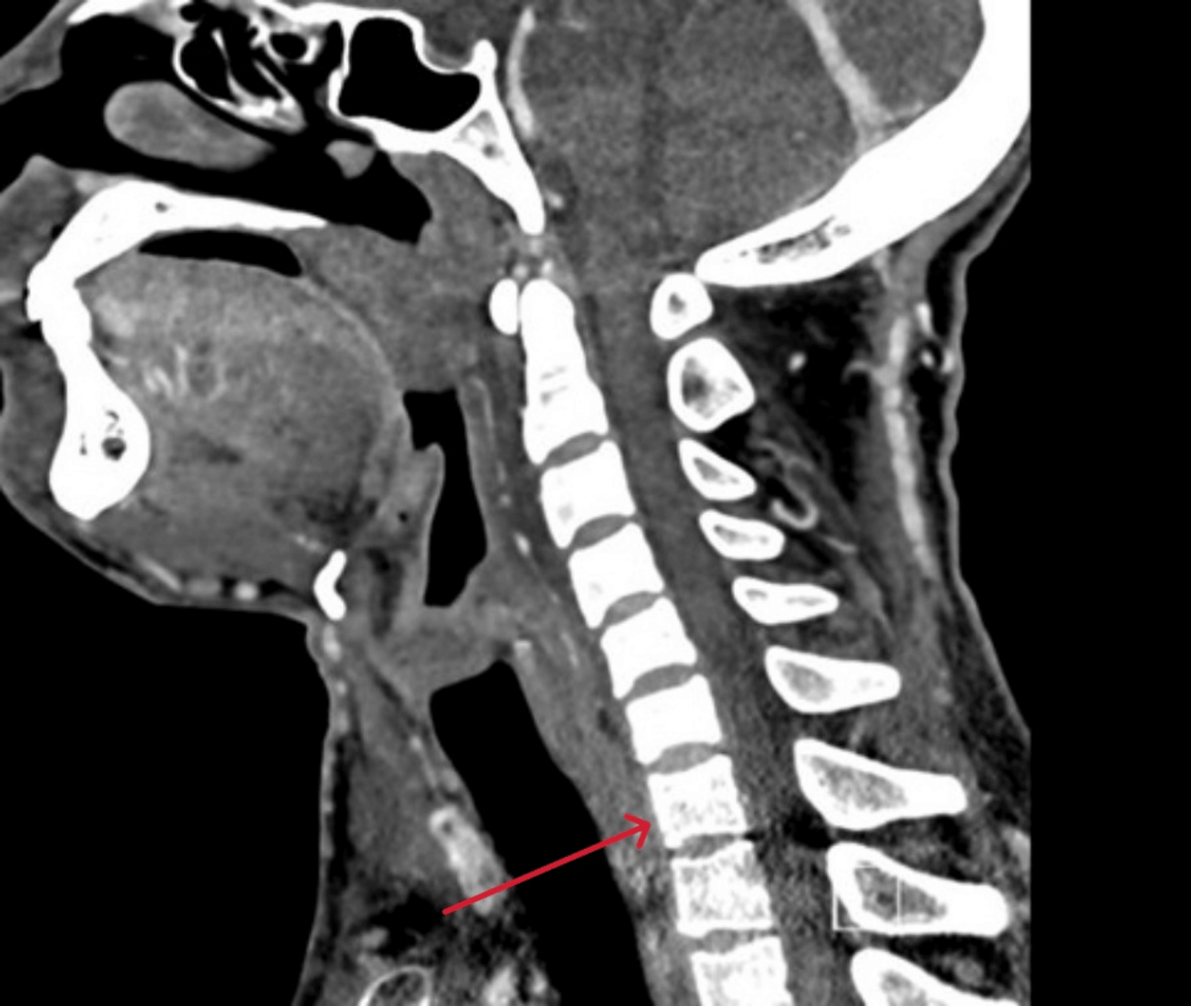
Computed tomography scan (coronal view) showing small prevertebral soft tissue edema at the C5-C6 level (red arrow)
